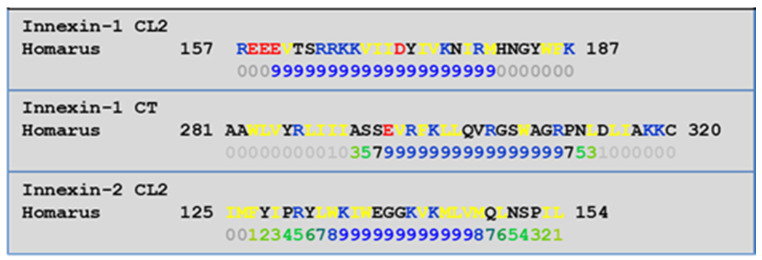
Innexins’ CaM-binding sites at CL2 and CT domain.
